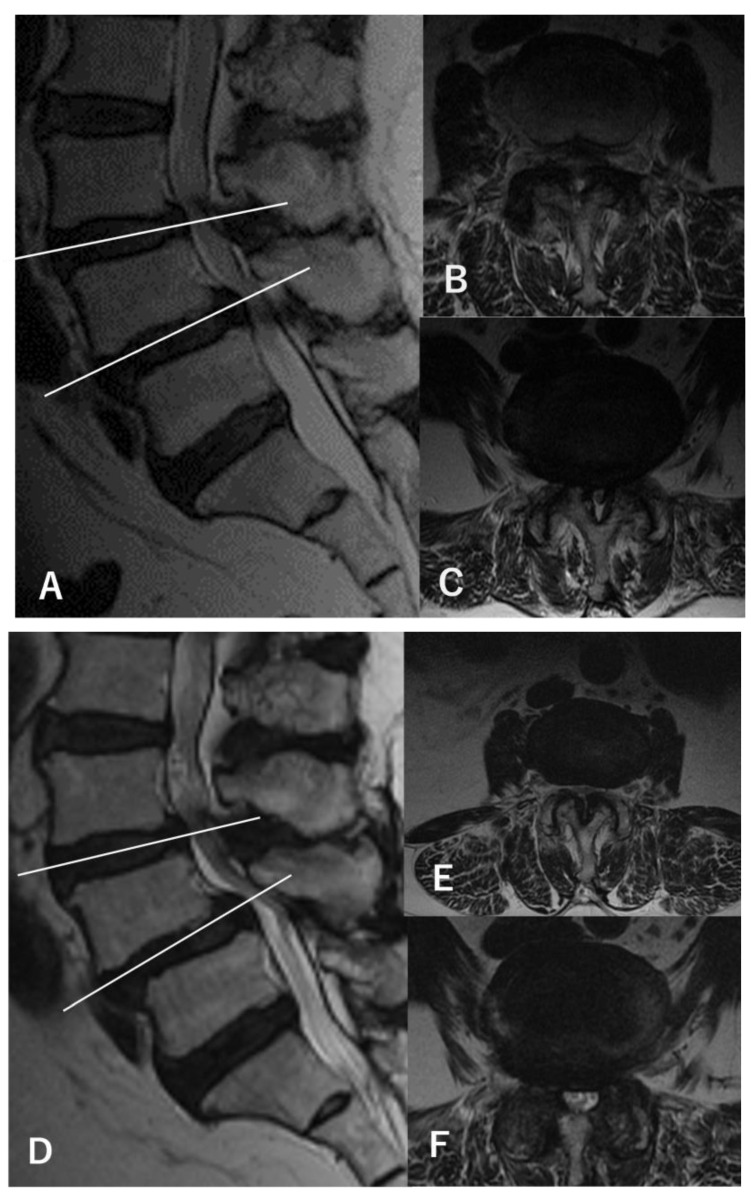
MRI findings of a representative case (a 67-year-old woman). (A) Preoperative sagittal-view MRI shows L3–4 and L4–5 stenosis with L4 spondylolisthesis. (B) The axial view of L3–4 shows a dural sac cross-sectional area (DSCA) of 42.6 mm^2^. (C) The axial view of L4–5 shows a DSCA of 28.3 mm^2^. The patient showed only bilateral L5 radiculopathy without any L4 findings during walking and standing load tests, and L4–5 decompression was performed using a microendoscope (microendoscopic laminectomy; MEL). After surgery, the symptoms disappeared, and no recurrences were observed within 5 years. Postoperative MRI at 5 years (D–F) shows only decompression at L4–5, with an L3–4 DSCA of 34.1 mm^2^ (E) and an L4–5 DSCA of 84.1 mm^2^ (F).
